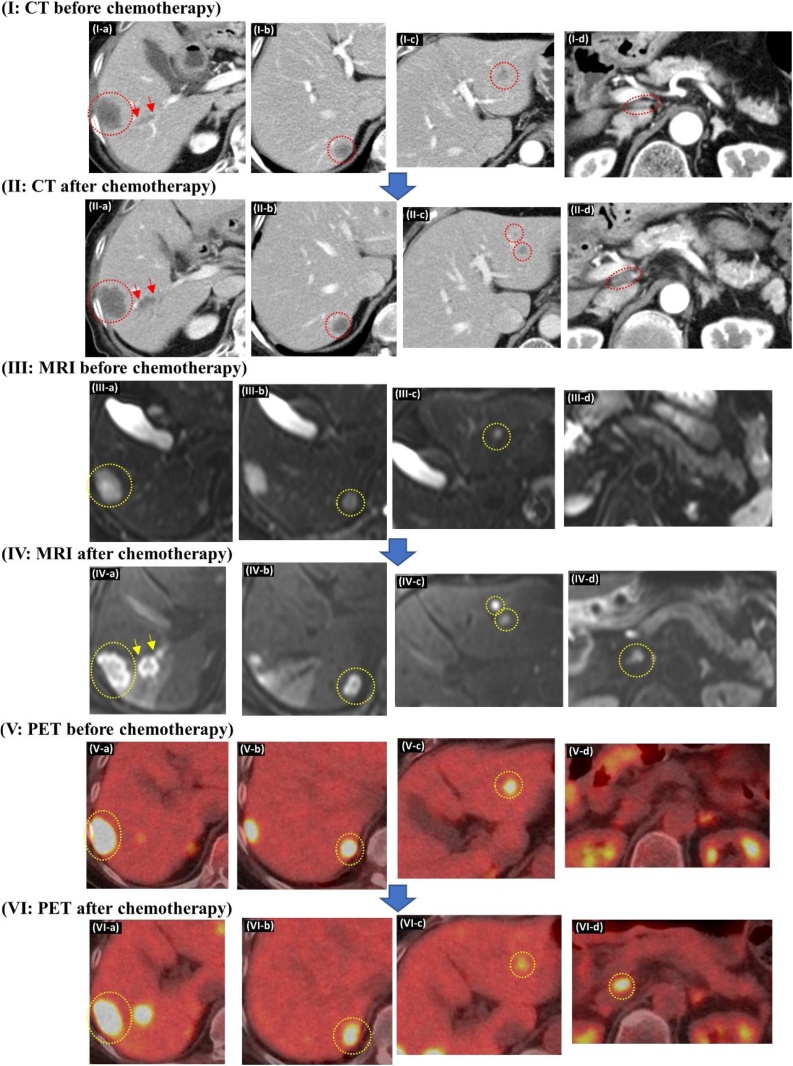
I-a, b, c) Three liver metastatic lesions (S6: size 35 mm, S7: size 23 mm, S3: size 13 mm). In addition, suspected tumor progression is seen along Glisson 6 (red arrow). I-d) Small LN detected in the hepatoduodenal ligament. II-a, b, c) In addition to the previous three liver metastatic lesions, one more metastasis is detected in S3. Furthermore, suspected tumor progression along Glisson 6 has become more evident (red arrow). II-d) LN in the hepatoduodenal ligament have increased in size when compared to the CT before chemotherapy. III-a, b, c, d) DWI-MRI displayed high intensity signals consistent with liver metastatic lesions. However, no signal intensity has been detected in the hepatoduodenal LN. IV-a, b, c) DWI-MRI shows high intensity in not only all the liver metastatic lesions including the new S3 metastasis, but also in Glisson 6 and the hepatoduodenal LN (yellow arrow). V-a, b, c, d) PET revealing high intensity signal consistent with all the liver metastatic lesions and Glisson 6, but not in the hepatoduodenal LN. VI-a, b, c, d) PET shows high intensity signals not only in all the liver metastatic lesions, but also Glisson 6 and the hepatoduodenal LN.
